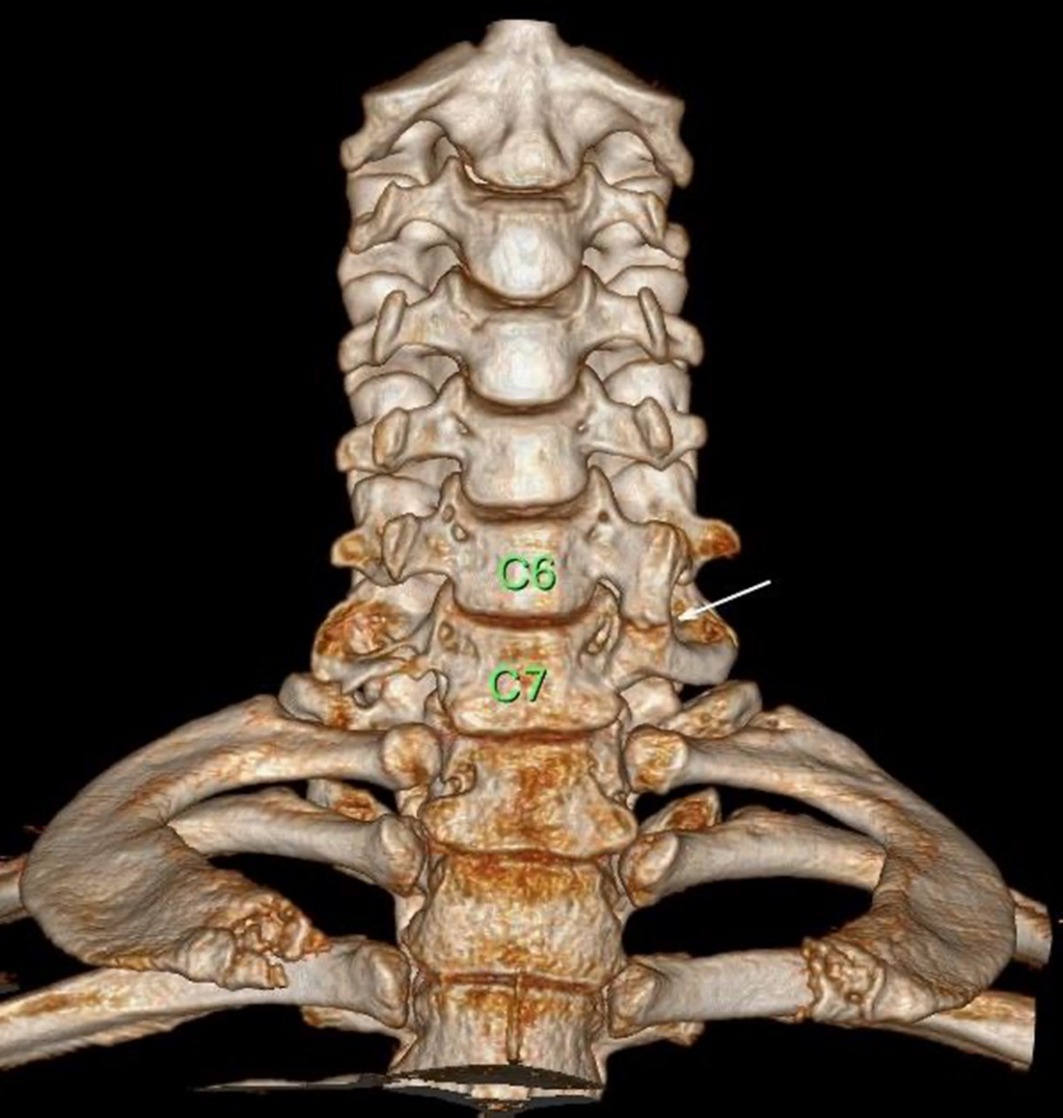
Coronal volume-rendered CT images best delineates the anatomy and clearly shows the accessory articulation between the C6/C7 transverse processes distinguishing it from an acute fracture.
